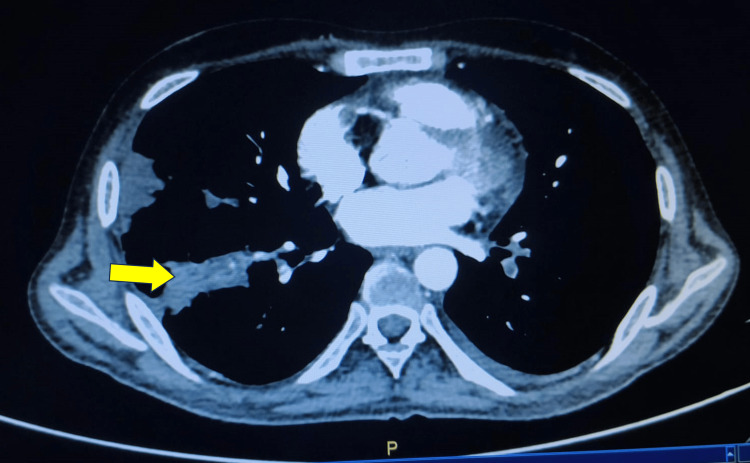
Computed tomographic pulmonary angiography demonstrating segmental thromboembolism in the right lower lobe pulmonary artery (yellow arrow)
